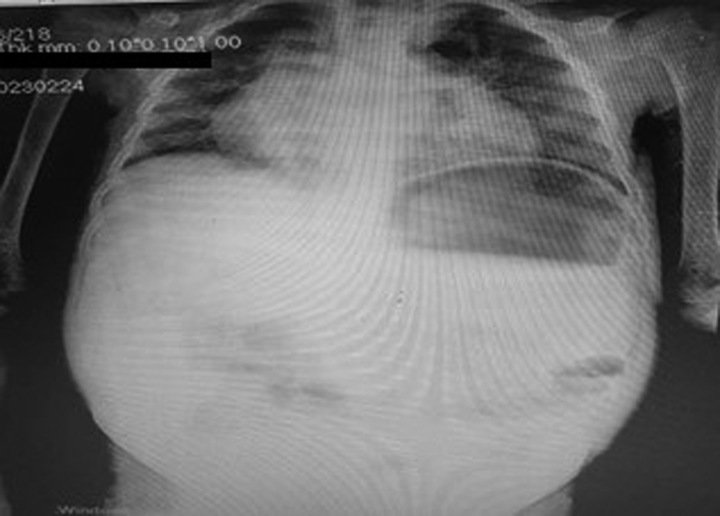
Chest X-ray showed severe bilateral infiltrates and cardiomegaly.
